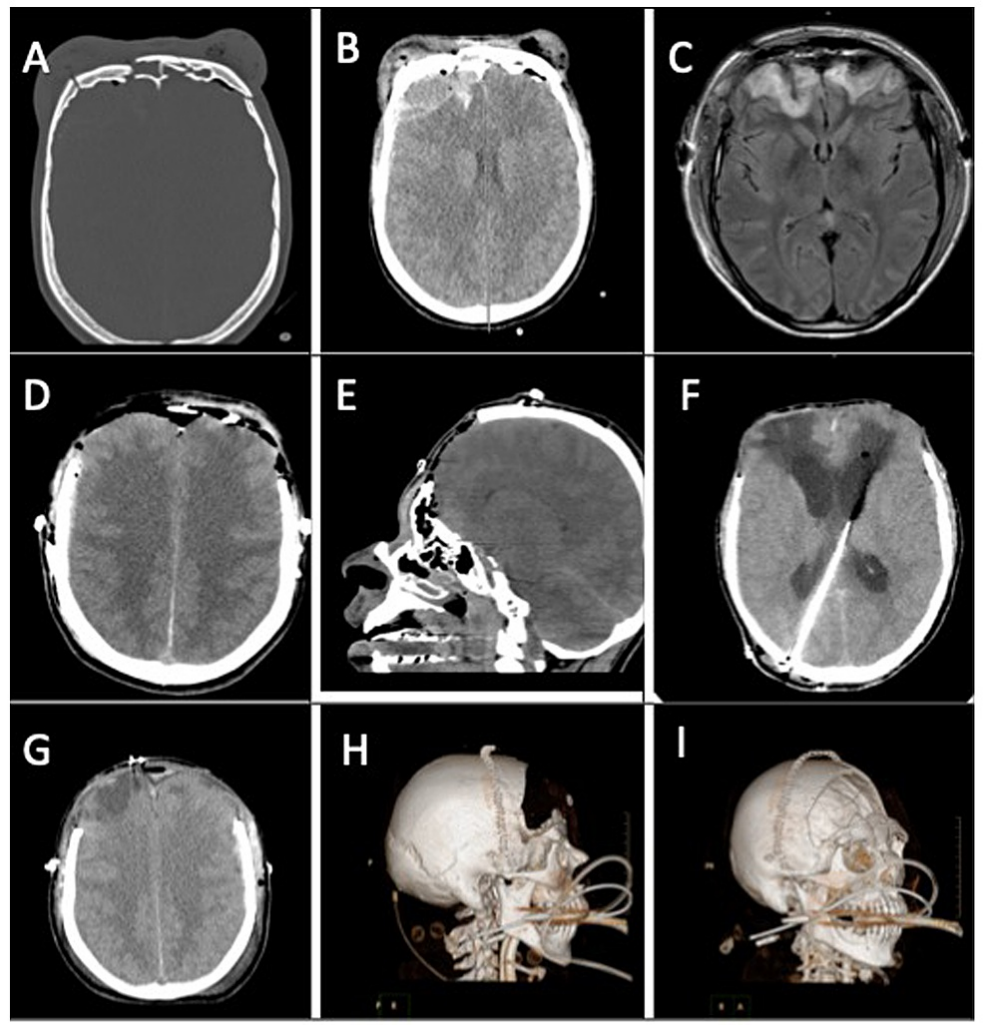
Neuroimaging findings of Case #1 A-B: CT of the head revealing extensive comminuted bilateral frontal bone fractures that violate the anterior and posterior walls of the frontal sinuses as well as a right frontal epidural hematoma, C: MRI of the brain revealing bilateral frontal lobe T2 FLAIR hyperintensities indicative of bifrontal contusions, D-E: Postoperative CT of the head following bifrontal decompressive craniectomy, F: Postoperative CT of the head following placement of a right occipital ventriculoperitoneal shunt, G-I: Postoperative CT of the head and 3-D image reconstruction following cranioplasty
